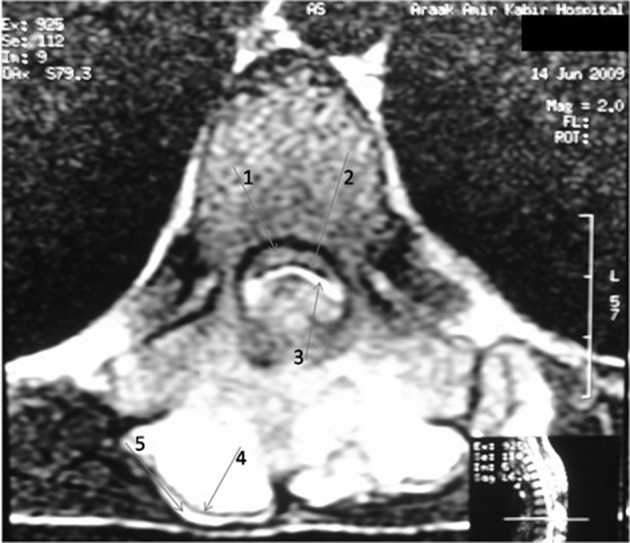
Axial T2-weighted MRI at the same level of Figure 3. Note the abnormal signal of the thinned and compressed cord (1), the displaced ligamentum flavum (2), the cartilage cap (3, 5) and the cortical bone layer (4)
